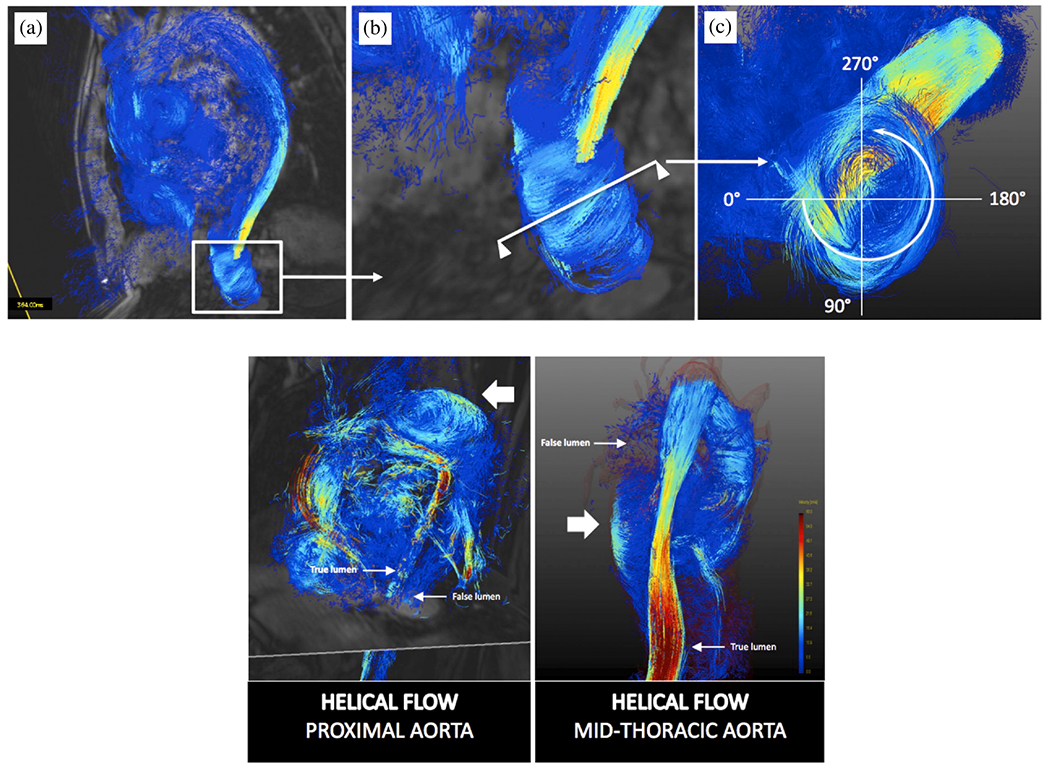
Visualization and quantification of helical flow in a patient with type B dissection assessed with 4D PC-MRI: (a) entire aorta examined to identify regions exhibiting helical flow, (b) plane placed perpendicular to the primary flow direction, and (c) helicity (in degrees per second) calculated by evaluating the amount of in-plane rotation and the start and end times of the cardiac cycle. Figure is reprinted from Clough et al. [158] with permission from Elsevier.
